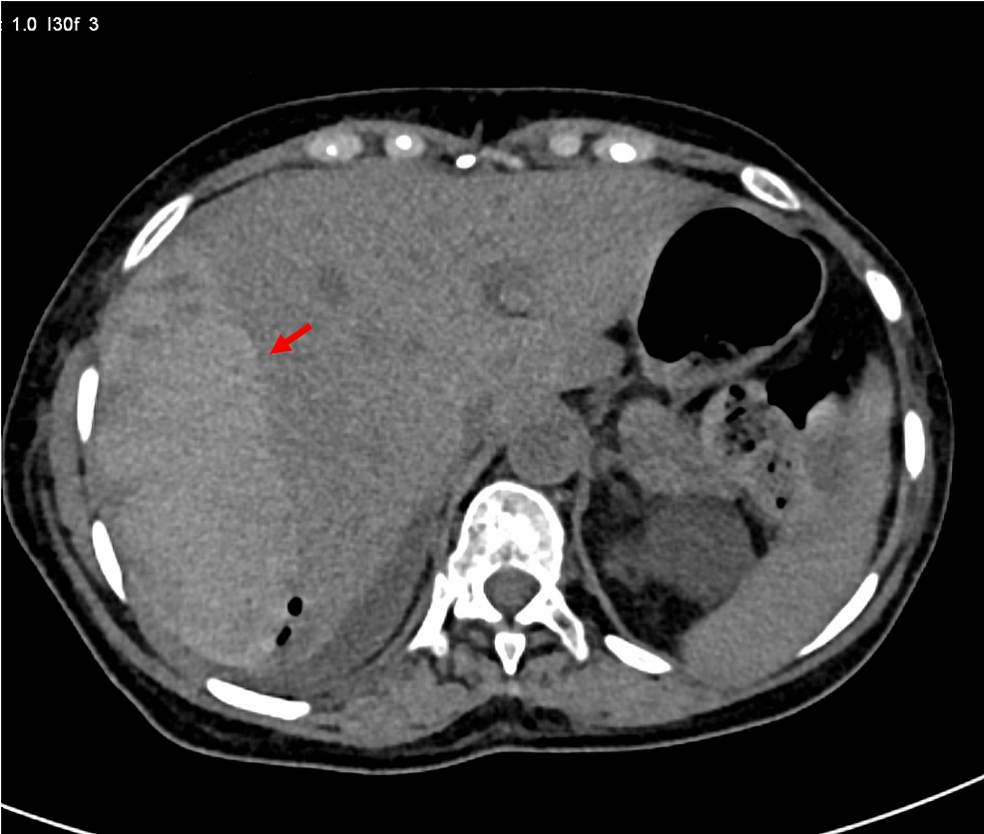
Axial non-contrast CT image showing the hyperdense subcapsular fluid collection indicating hematoma (arrow)
